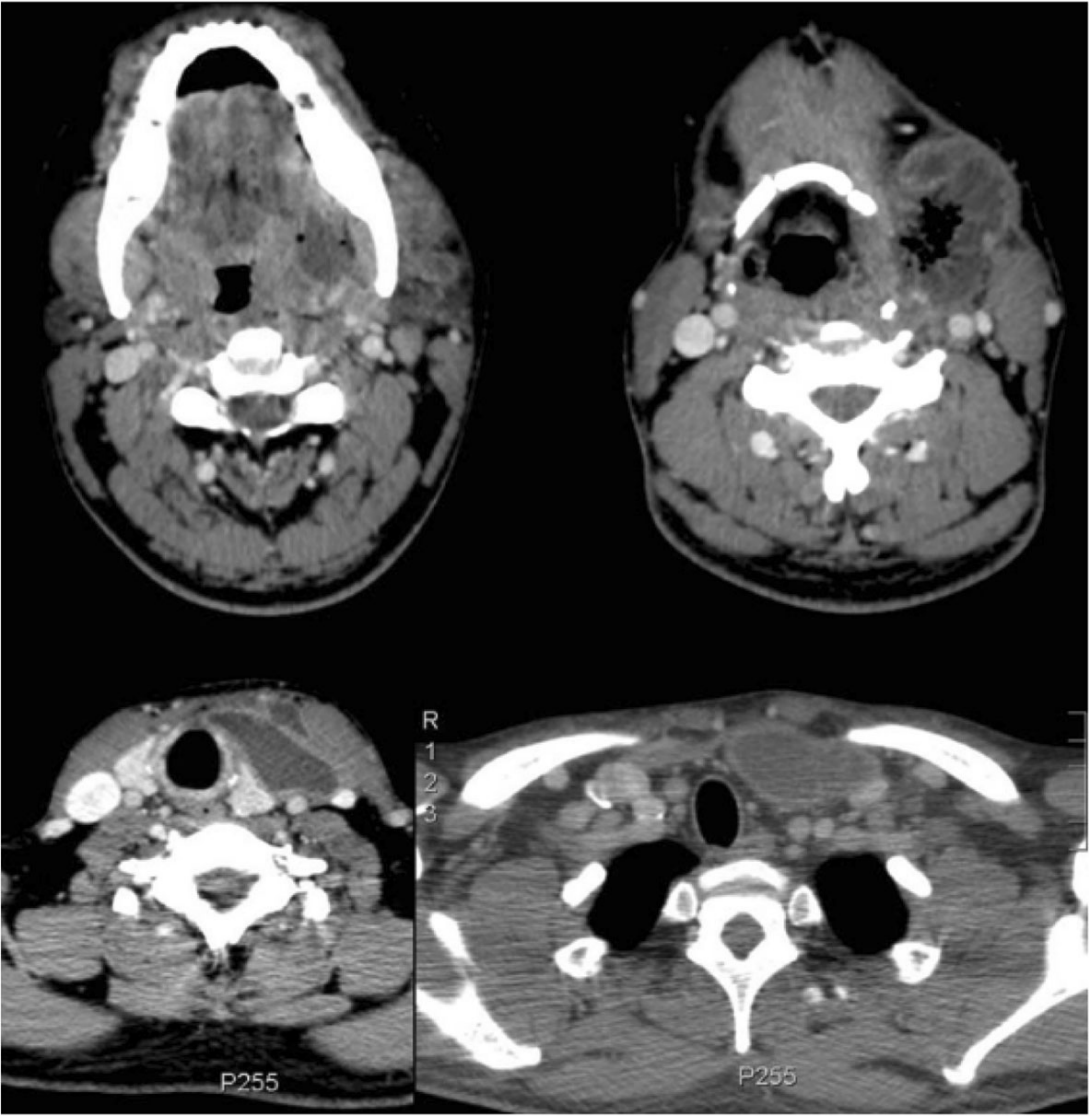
Computed tomography of the neck with evidence of extension of the left collection affecting the retromandibular, submandibular, parapharyngeal, and vascular spaces, as well as the upper mediastinum
